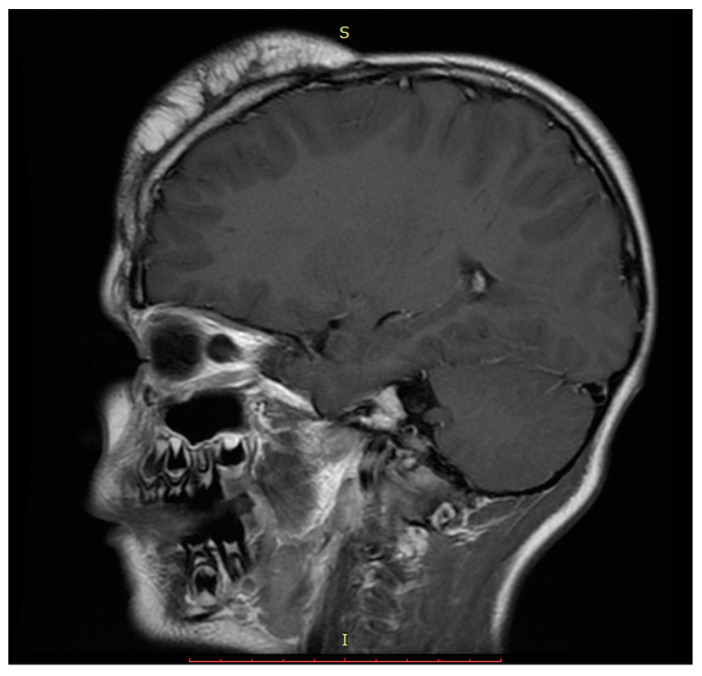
Sagittal T1 magnetic resonance image showing heterogenous lobulated fat in the high frontal scalp.
